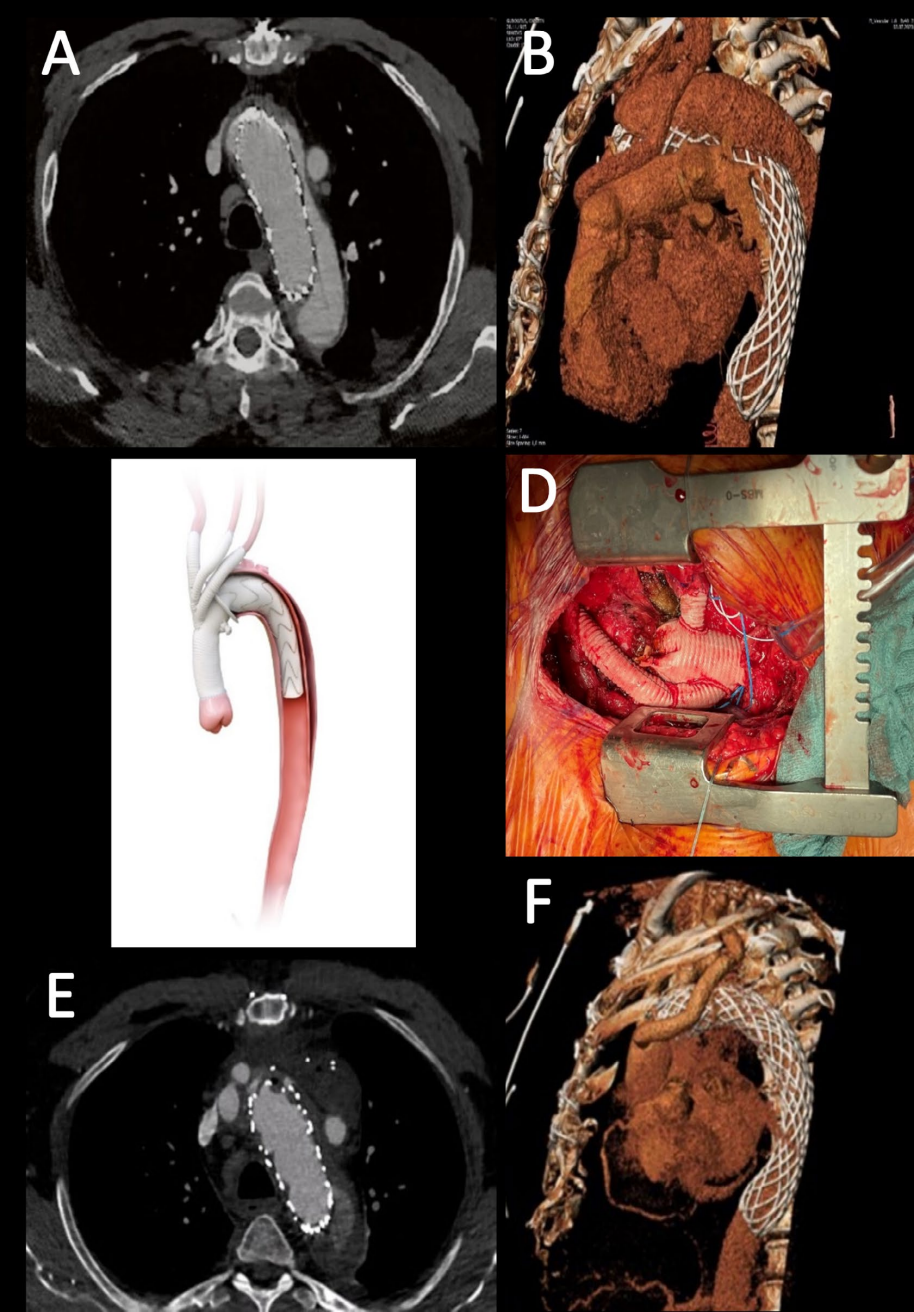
Redo surgery with minimally invasive FET using E-Vita OPEN NEO trifurcated after failed AMDS. (A) Preoperative computed tomographic angiography (CTA) revealing an enlarged aortic arch post AMDS implantation with a patent false lumen (FL). (B) Preoperative CT 3D reconstruction of AMDS prosthesis. (C) The new E-vita OPEN NEO Trifurcated (Permission: Artivion, Inc.). (D) Implanted E-vita OPEN NEO trifurcated with debranched supra-aortic vessels via upper ministernotomy. (E) Postoperative CTA showing optimal perfusion of supra-aortic vessels and closure of the FL. (F) Postoperative CTA 3D reconstruction demonstrating excellent stent-graft implantation and diminished FL diameter
